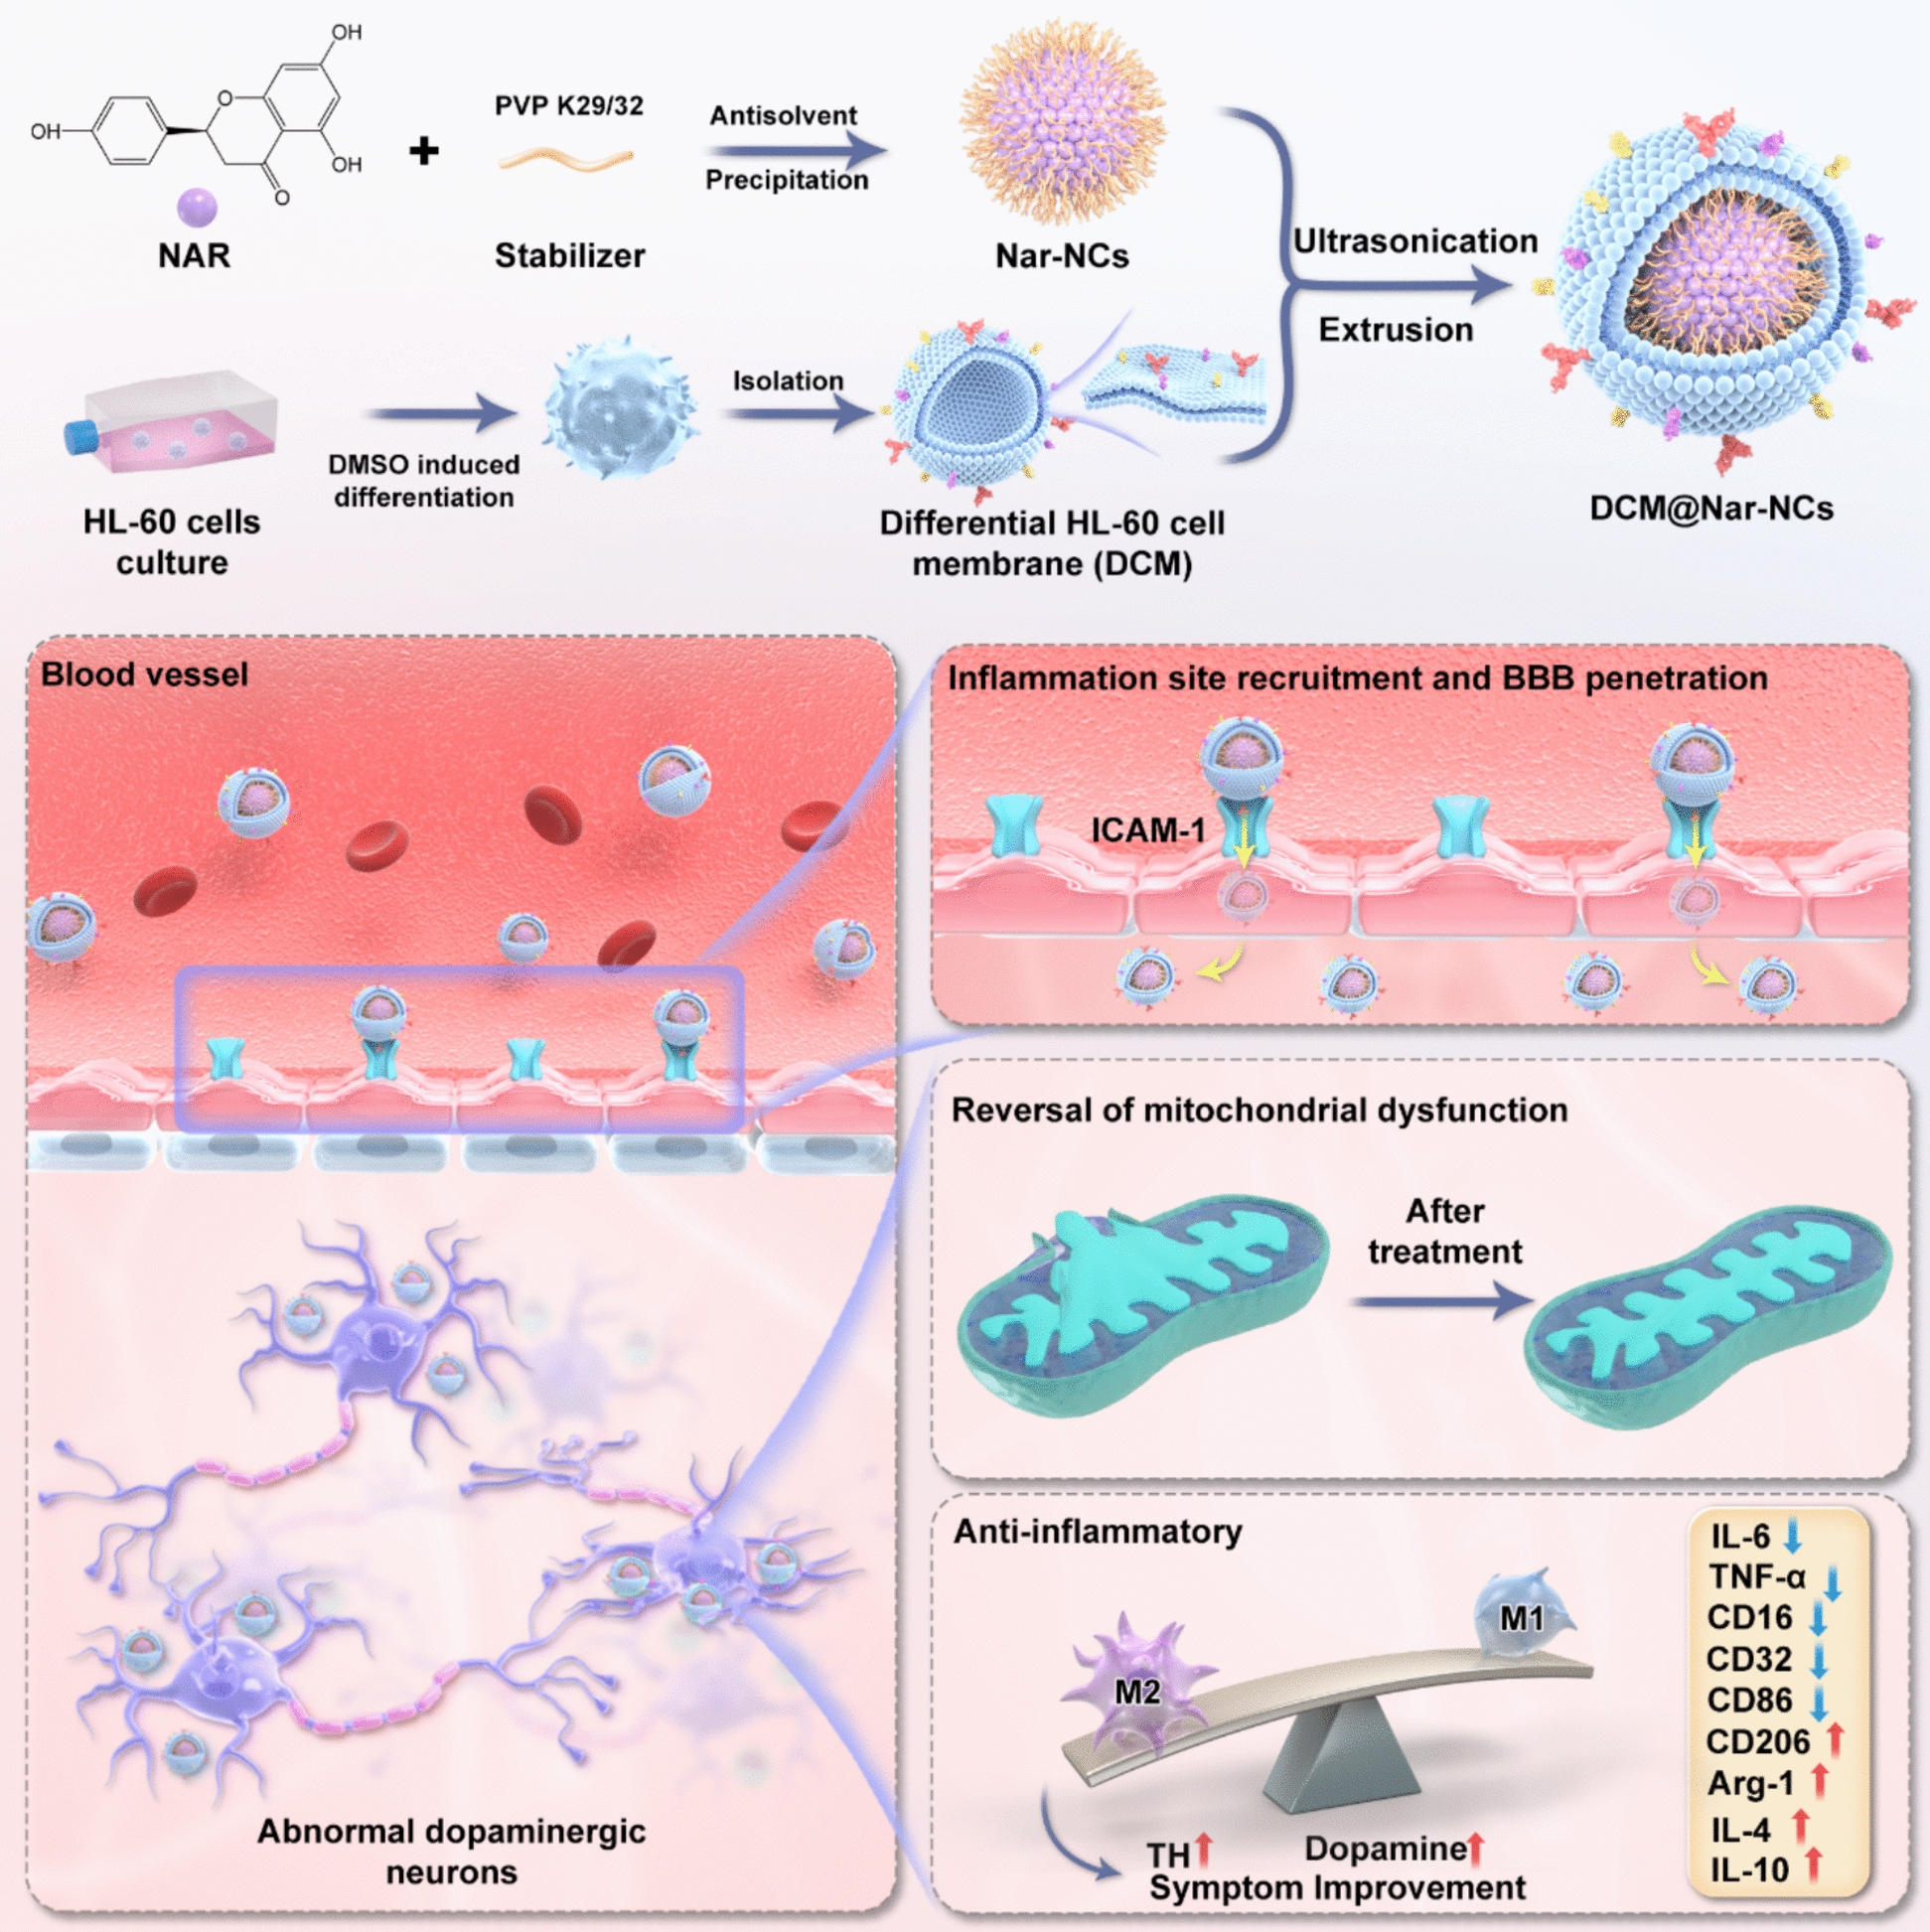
Synthesis and application of DCM@Nar-NCs for PD treatment. To prepare DCM@Nar-NCs, Nar-NCs were encapsulated within differentiated HL-60 cell membranes (DCMs). This was followed by ultrasonication and extrusion to generate the core–shell structure of DCM@Nar-NCs. Specifically, the surface of Nar-NCs was coated with DCM. The LFA-1 protein on the DCM surface could specifically bind to the ICAM-1 protein on the membranes of brain endothelial cells. This allowed the nanodelivery system to cross the BBB, target sites of inflammation, release drugs, and treat the pathological changes associated with PD. Treatment with DCM@Nar-NCs reversed mitochondrial dysfunction and ameliorated neuroinflammation, thereby improving motor function and alleviating PD-related symptoms in PD models
